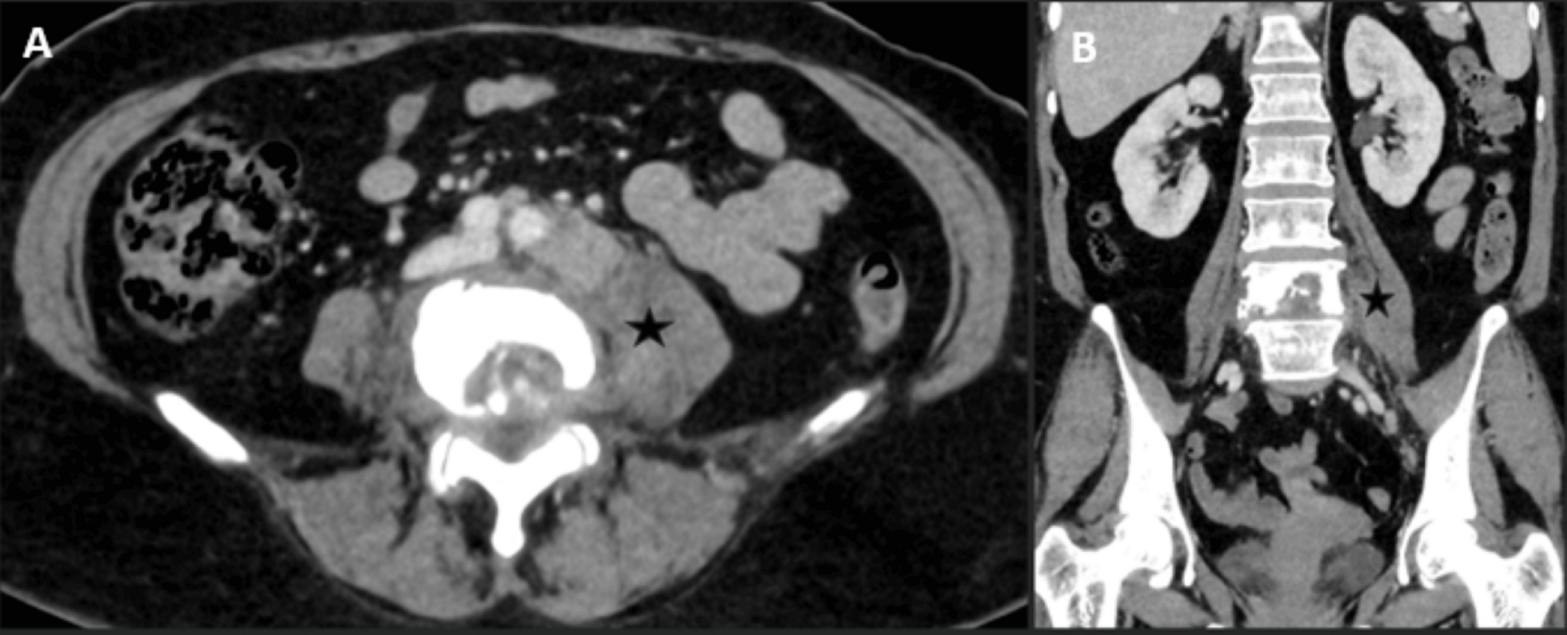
Axial and coronal images Axial (A) and coronal (B) images from a follow-up CT scan show a marked interval reduction in the size of the left psoas abscess (*). CT, Computed Tomography
